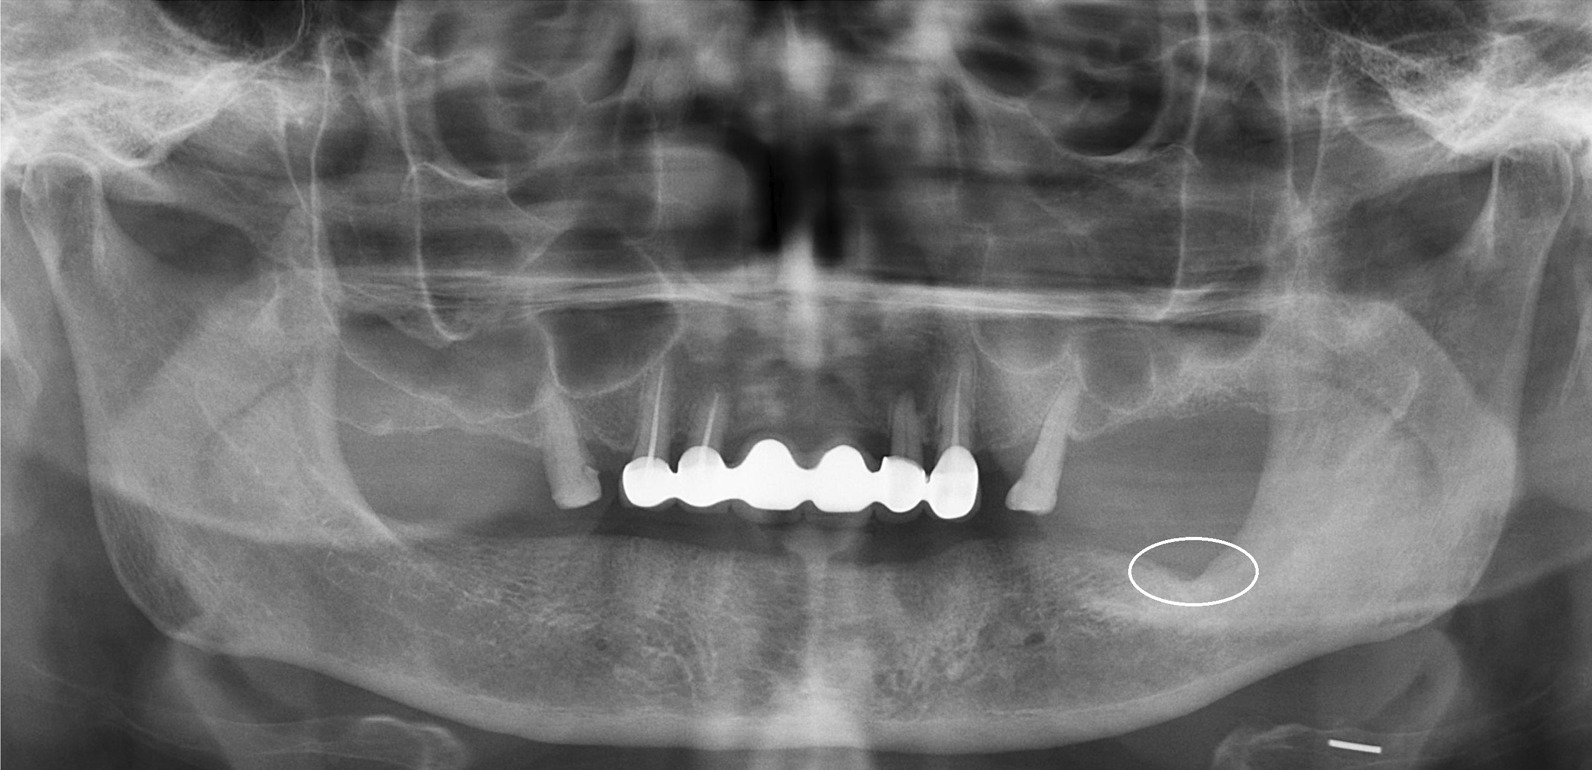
Postoperative panoramic radiograph of the patient showing arrest of disease progression and remodeling of the affected area at six months after surgery. Encircled area shows no sign of osteonecrosis or new sequestrum
